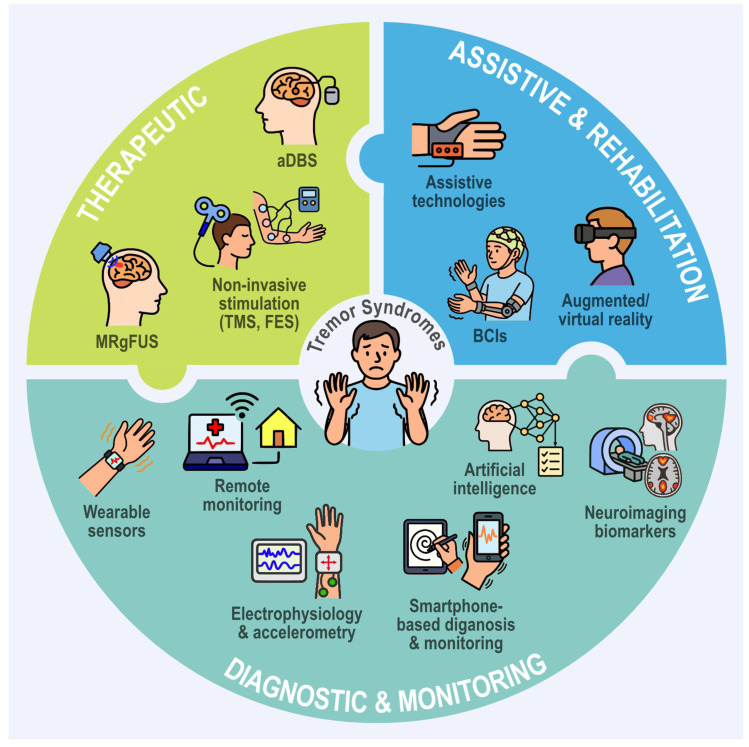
Technological innovations advancing tremor diagnosis and therapy. Emerging technologies are transforming tremor care by enabling more precise diagnoses, individualised therapies, and enhanced rehabilitation. Innovations are grouped into three main domains: therapeutic—encompassing adaptive deep brain stimulation (aDBS), magnetic resonance-guided focused ultrasound (MRgFUS), and non-invasive stimulation techniques such as transcranial magnetic stimulation (TMS) and functional electrical stimulation (FES); assistive and rehabilitation—including assistive devices, brain–computer interfaces (BCIs), and augmented or virtual reality platforms designed to enhance motor function and user engagement; and diagnostic and monitoring—covering wearable sensors, electrophysiology and accelerometery, smartphone-based tools, artificial intelligence applications, remote monitoring systems, and neuroimaging biomarkers. Together, these technologies support a multidimensional, personalised approach to tremor management in both clinical and home settings.
